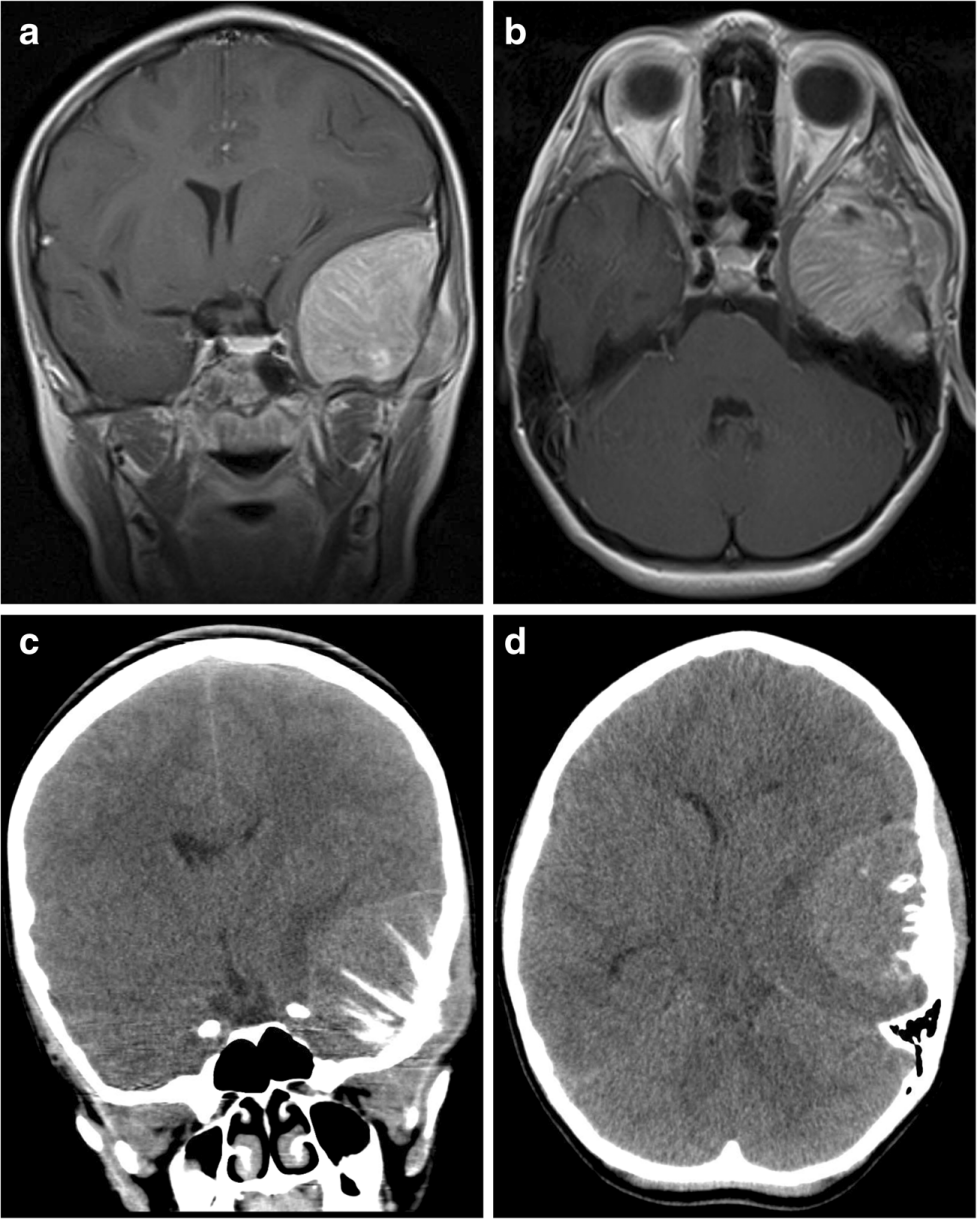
a Images from Case 2 of a 10-year-old female presenting with a left temporal lobe tumor. Extensive remodeling of the intracranial surface of the left temporal bone is observed. Coronal T1-weighted MRI with contrast. b Axial T1-weighted MRI with contrast. c Coronal non-contrast CT. d Axial non-contrast CT
